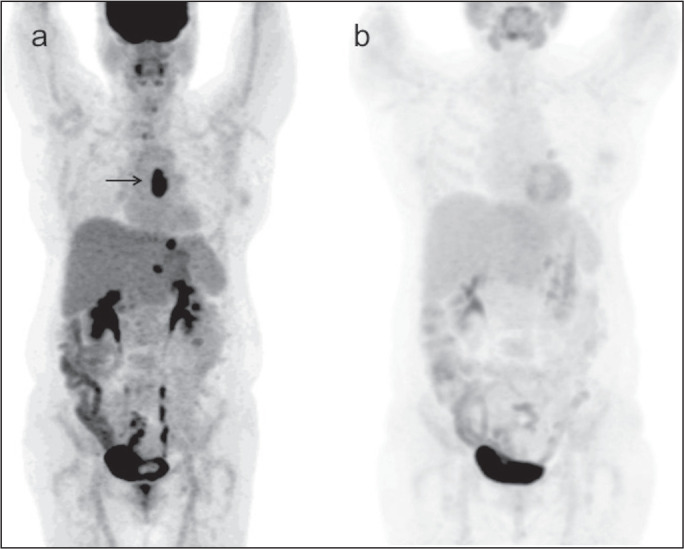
a: MIP image of the ^18^F-FDG-PET/CT scan
